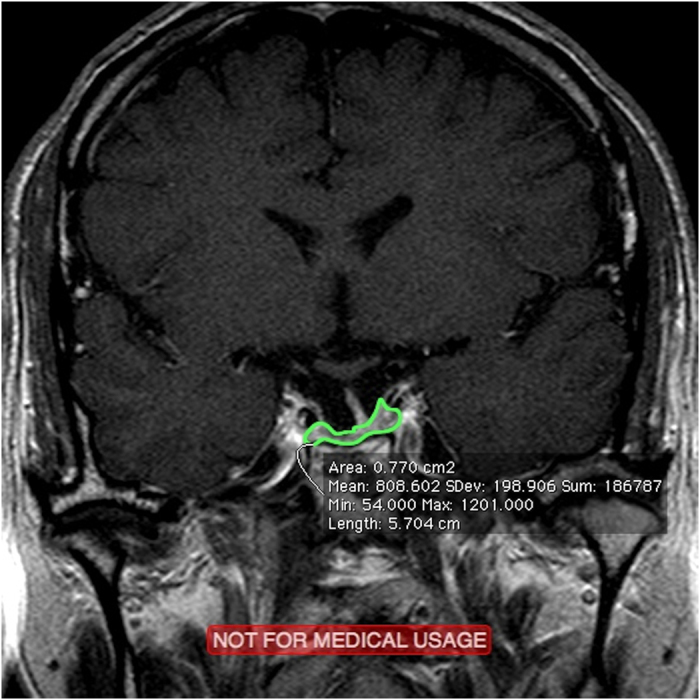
GTR status was achieved in a 60-year-old male patient, the postoperative MRI of whom revealed no tumor remnants but did exhibit a regularly shaped pituitary gland and granulation tissue (excluding the stalk; green area).
